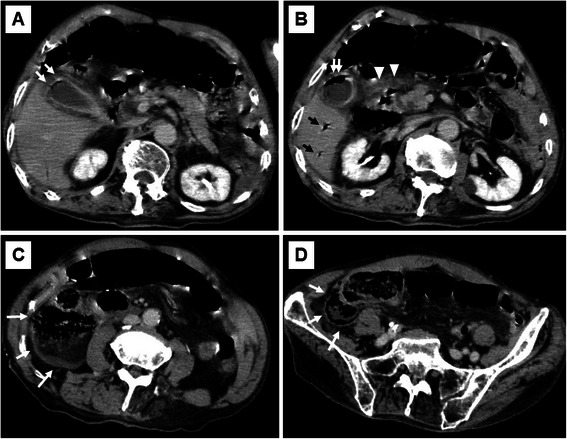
Abdominal CT findings. a: CT showed a thickened wall and intramural air of the gallbladder, suggesting emphysematous cholecystitis (white arrows). b: Pneumobilia was detected in the intrahepatic bile duct (black arrows). The gallbladder contained air within the lumen (white arrows). Subserosal free air was detected in the hepatoduodenal ligament and around the pancreas head (white arrowheads). c: Massive pneumoretroperitoneum was observed behind the right-sided colon (white arrows). d: Pneumoretroperitoneum extended to the retroperitoneal space behind the ascending mesocolon, bordered by the cecum and iliopsoas muscle (white arrows)
